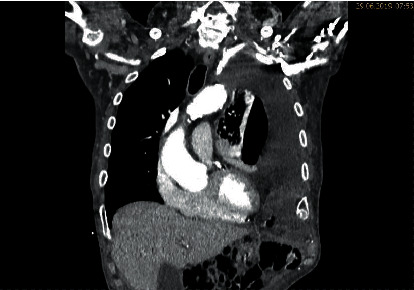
Coronary thoracoabdominal CT scan with left-sided seropneumothorax.
